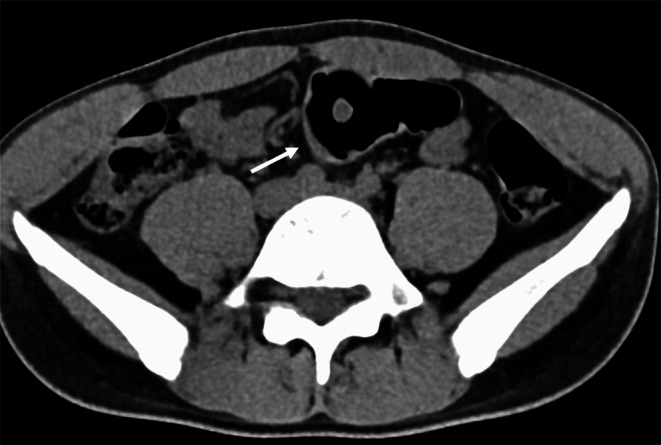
Axial contrast‐enhanced CT showing a cylindrical intrarectal foreign body (arrow) without surrounding inflammation or free air.
